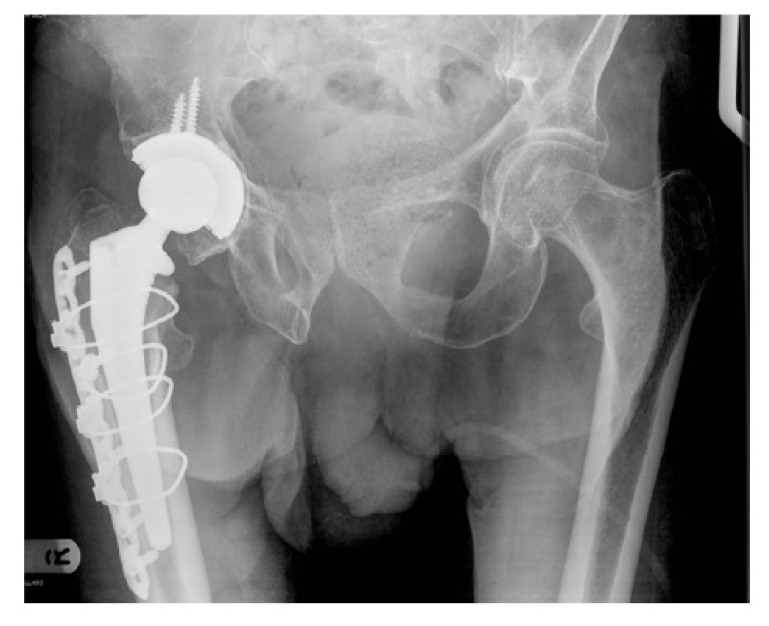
Anterio-posterior pelvic radiograph of Case 2 following the fall (a).
